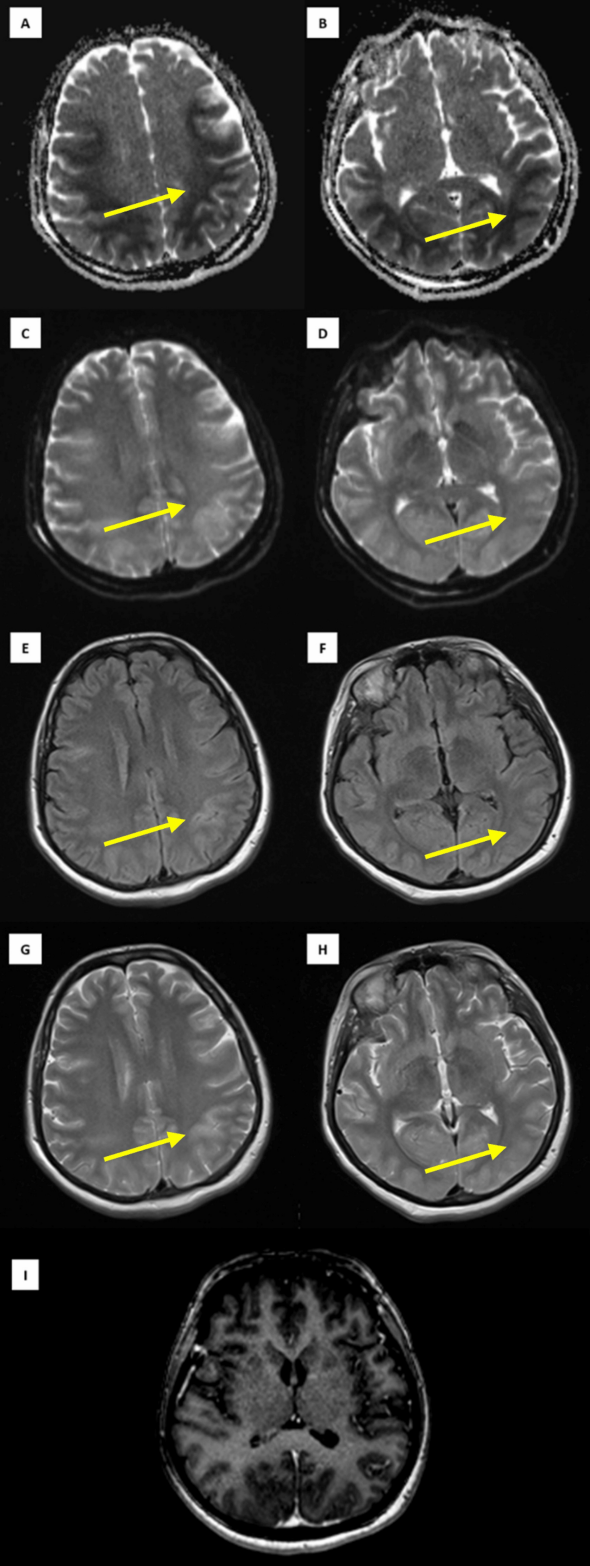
An MRI of the brain (axial) demonstrating bilateral fronto-parieto-occipital cortical and subcortical changes consistent with posterior reversible encephalopathy syndrome (yellow arrows). (A, B) Apparent diffusion coefficient (ADC) maps showing restricted diffusion in the white matter; (C, D) Corresponding bright signals on diffusion-weighted imaging (DWI); (E, F) Fluid-attenuated inversion recovery (FLAIR) images; (G, H) T2-weighted imaging (T2WI) revealing bilateral hyperintense cortical lesions; (I) Contrast-enhanced T1-weighted imaging (T1W C+) showing no postcontrast enhancement; the cortex appears diffusely expanded bilaterally, with no notable changes in the cerebellum, thalamus, basal ganglia, or brainstem.
